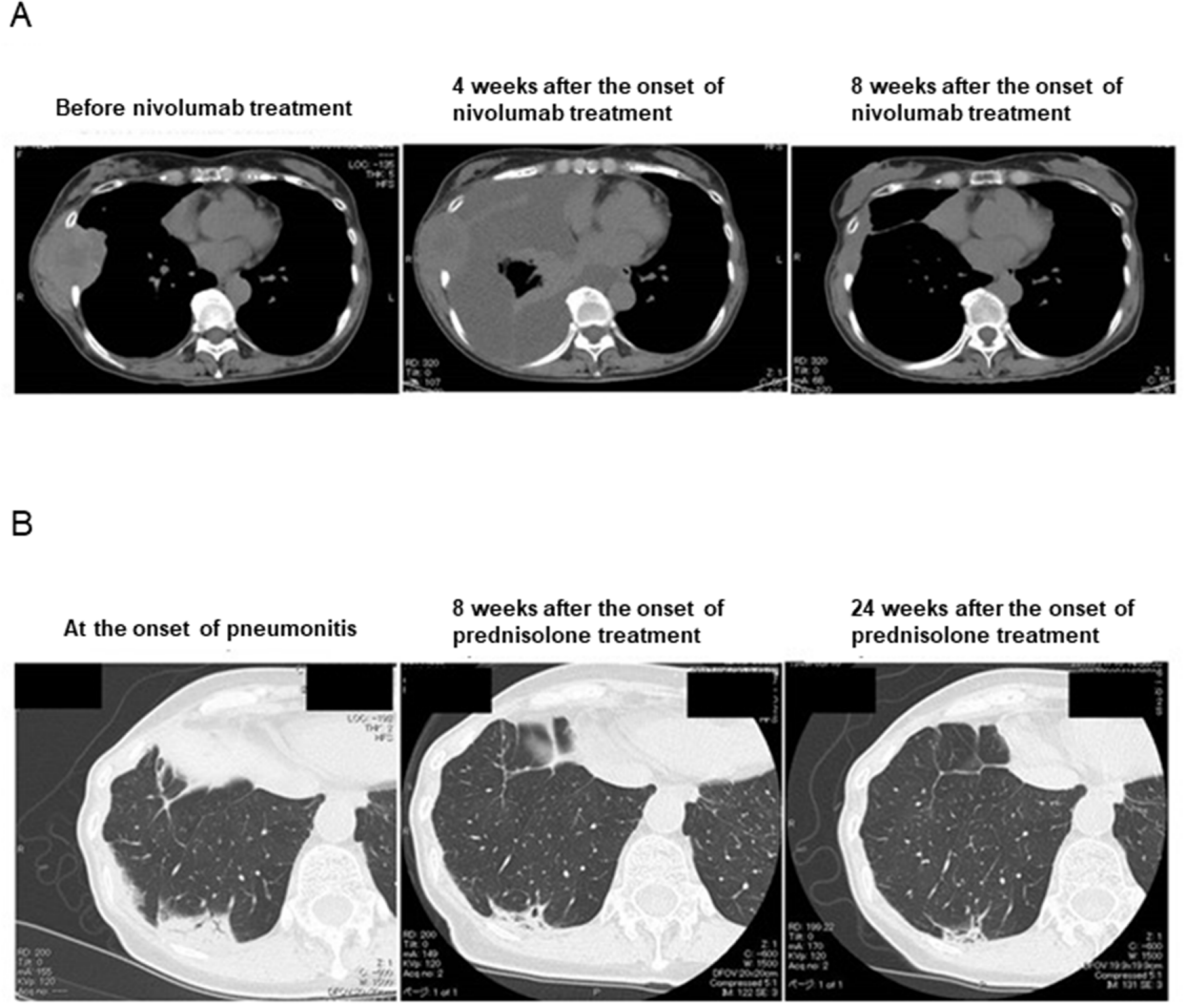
Chest CT scans of the patient (A) Clinical course of the pleural effusion and metastatic tumors. (B) Clinical course of the pneumonitis.
